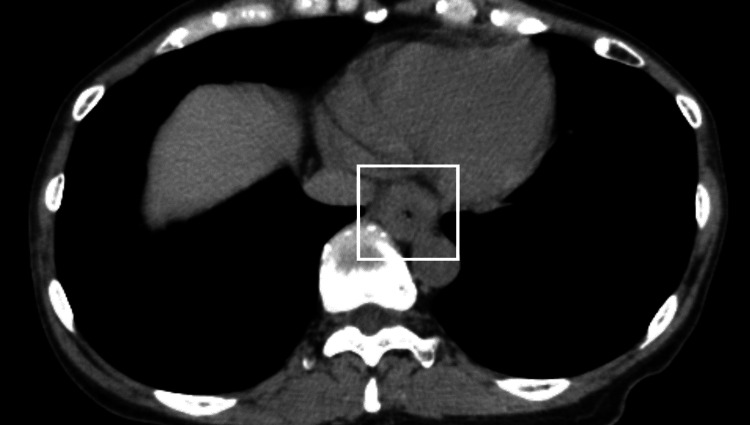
Chest computed tomography image of the lower esophagus Chest CT showed circumferential wall edema from the middle to the lower esophagus (□).
